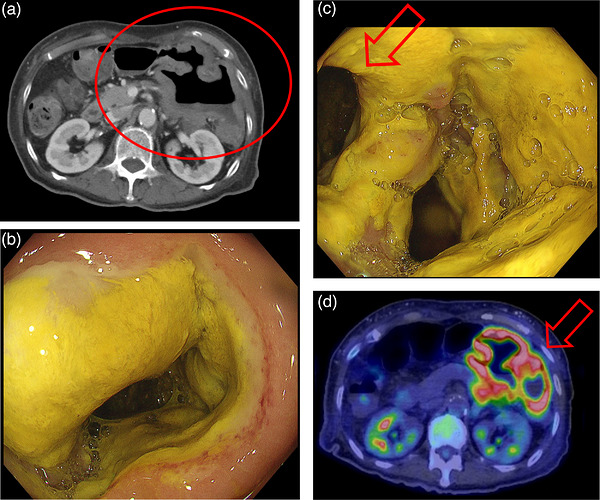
Contrast‐enhanced CT, colonoscopy, and PET–CT findings. (a) Contrast‐enhanced CT demonstrated marked wall thickening and dilatation extending from the splenic flexure to the descending colon. Wall thickening of the proximal jejunum near the ligament of Treitz was also noted, suggesting continuity between the jejunal and colonic lesions (circles). (b and c) Colonoscopy showed a circumferential ulcerative lesion at the splenic flexure. Proximal to the lesion, an additional lumen deviating from the normal colonic lumen was identified (arrows), suggesting the presence of an internal fistula. The lumen at the tumor site was dilated, raising suspicion of aneurysmal‐type malignant lymphoma. (d) PET–CT revealed abnormal FDG uptake in the splenic flexure and descending colon, as well as in the horizontal portion of the duodenum and proximal jejunum (arrows).
